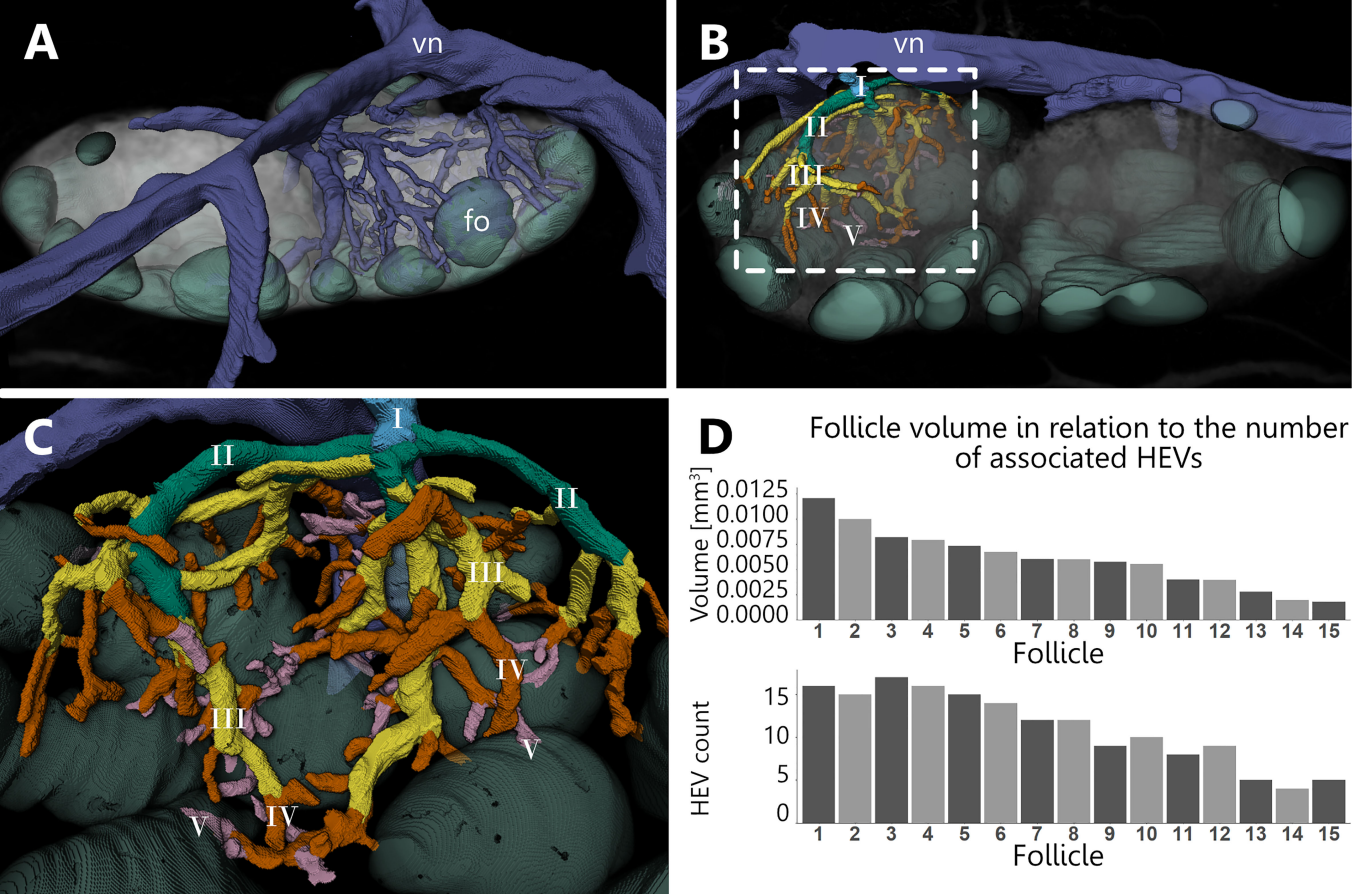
Branching pattern of the venous system and high endothelial venule (HEV) count. An animated visualization of this figure is available in the supplementary files (Supplement Video S4). (A–C) Volume rendering of an image stack acquired by means of X-ray phase-contrast imaging with a 2D Talbot array. (A) Anterior view. Overview on the distribution of the venous system for lobe I (see Figure 5). The venous system projects throughout all compartments despite the follicles. (B) Posterior view. Branching pattern of one of the main arches of the venous system is color coded, resembling five different orders (I-V). Venules of the order III to V are corresponding to HEVs which are often seen closely associated with B-cell follicles. (C) Close-up view on the branching pattern depicted in panel (B, D) For each follicle from lobe I, the total count of HEVs was determined which drain the corresponding capillary system of the follicles. With decreasing follicle volumes, the individual HEV counts show a declining trend. B-cell follicle=fo (mint green), venous system=vn (blue).
